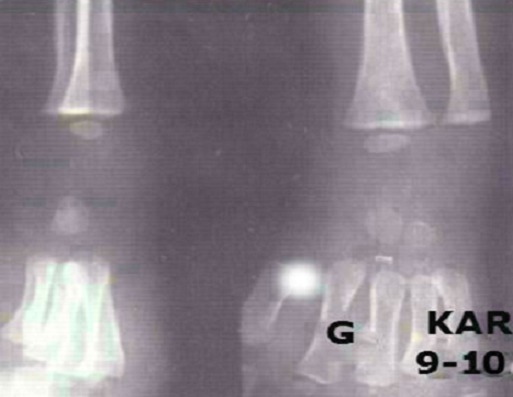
La radiographie initiale du poignet après traumatisme immediate
